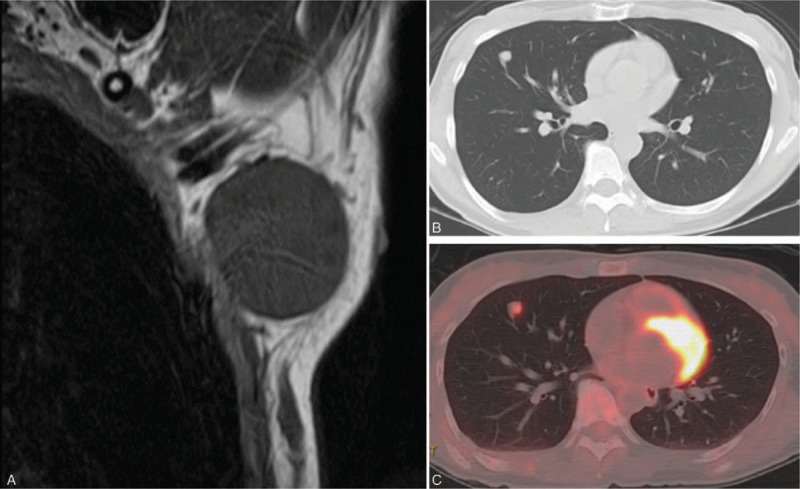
(A) Magnetic resonance imaging revealed a clearly distinct tumor and a low signal in the T1-weighted image. (B) Chest computed tomography showed a 10 × 10 mm nodule in the right S4 area. (C) Positron emission tomography-computed tomography showed slight uptake in the nodule, with a maximal standardized uptake value of 2.94.
